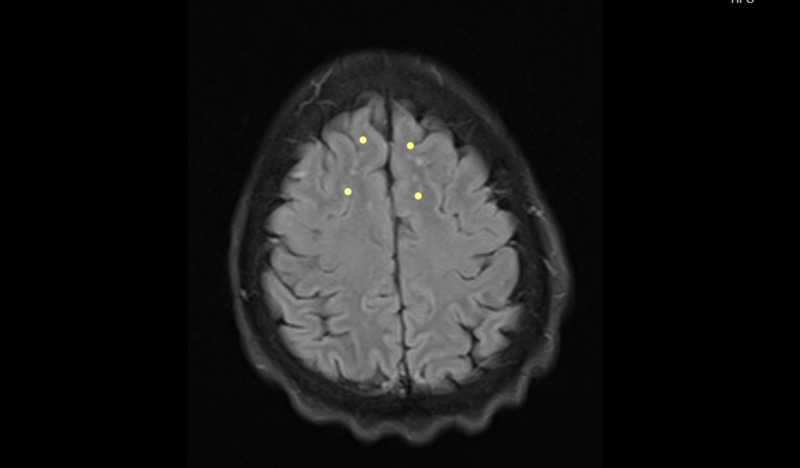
Brain MRI - view 3 FLAIR axial and T2-weighted image. The image shows hyperintensity in the path of the corticospinal tract (yellow dots) MRI: magnetic resonance imaging; FLAIR: fluid attenuation inversion recovery
